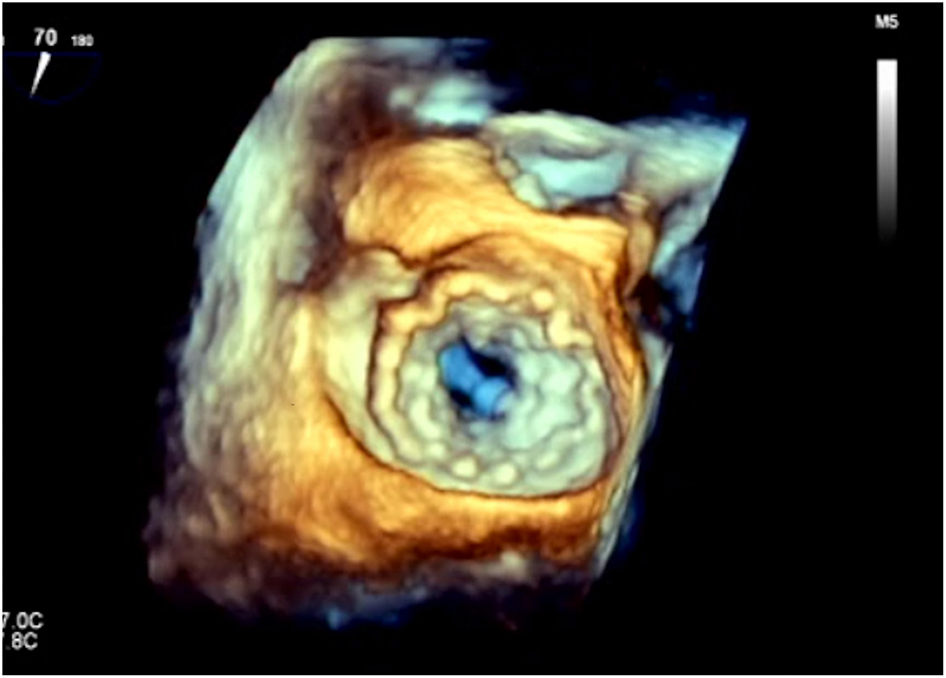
3D transesophageal echocardiography showing the atrial side of a Tendyne^TM^ valve (Abbott) after deployment. It exhibits the huge artificial anchoring surface of these devices.
